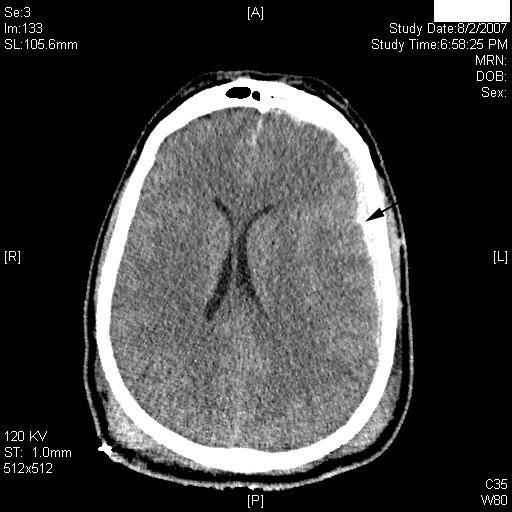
Computed tomography scan at the level of the lateral ventricles. There is a thin layer of acute subdural hematoma identified on the left with a minimal midline shift (arrow). A = anterior; P = posterior; L = left; R = right.
